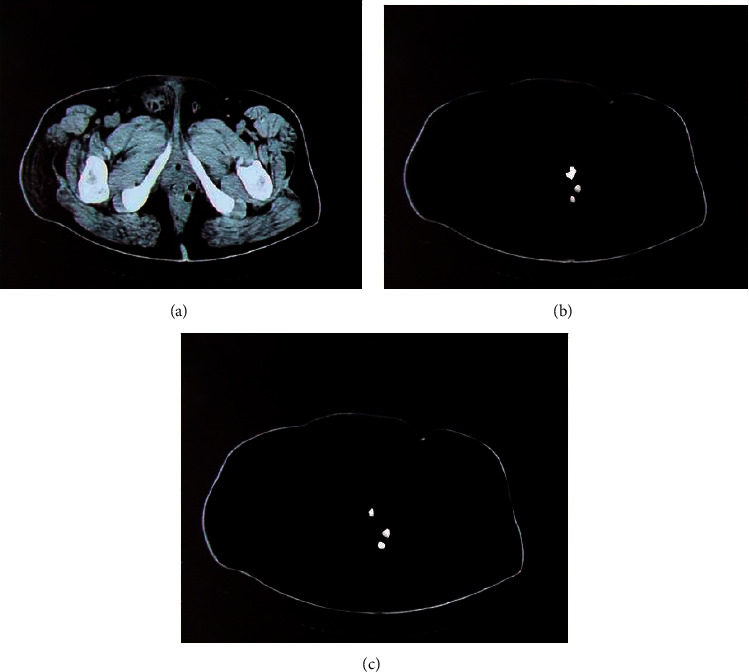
Comparison on the segmentation effect of the two algorithms. (a) Input CT image of perianal abscess tissue. (b) Segmentation effect of CNN algorithm. (c) Segmentation effect of DLFCNN algorithm.
